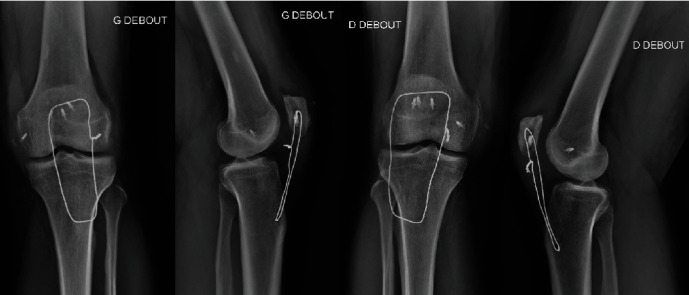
Radiographic control 10 weeks after surgery.
